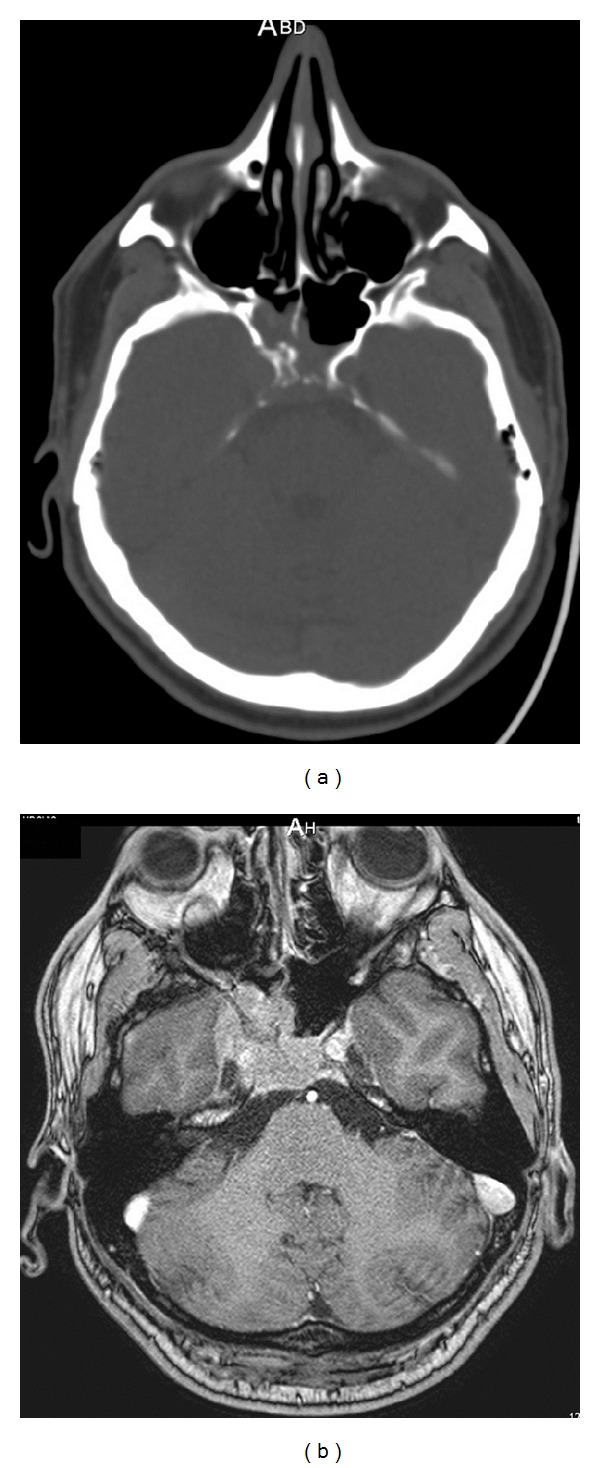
(a) Enlargement of the right cavernous sinus and osteolysis of the right sphenoid bone and clivus on CT scan. (b) Axial T1-weighted MRI with gadolinium contrast showing a large tumor of the skull base invading the sella turcica, right cavernous sinus, and sphenoidal sinus.
